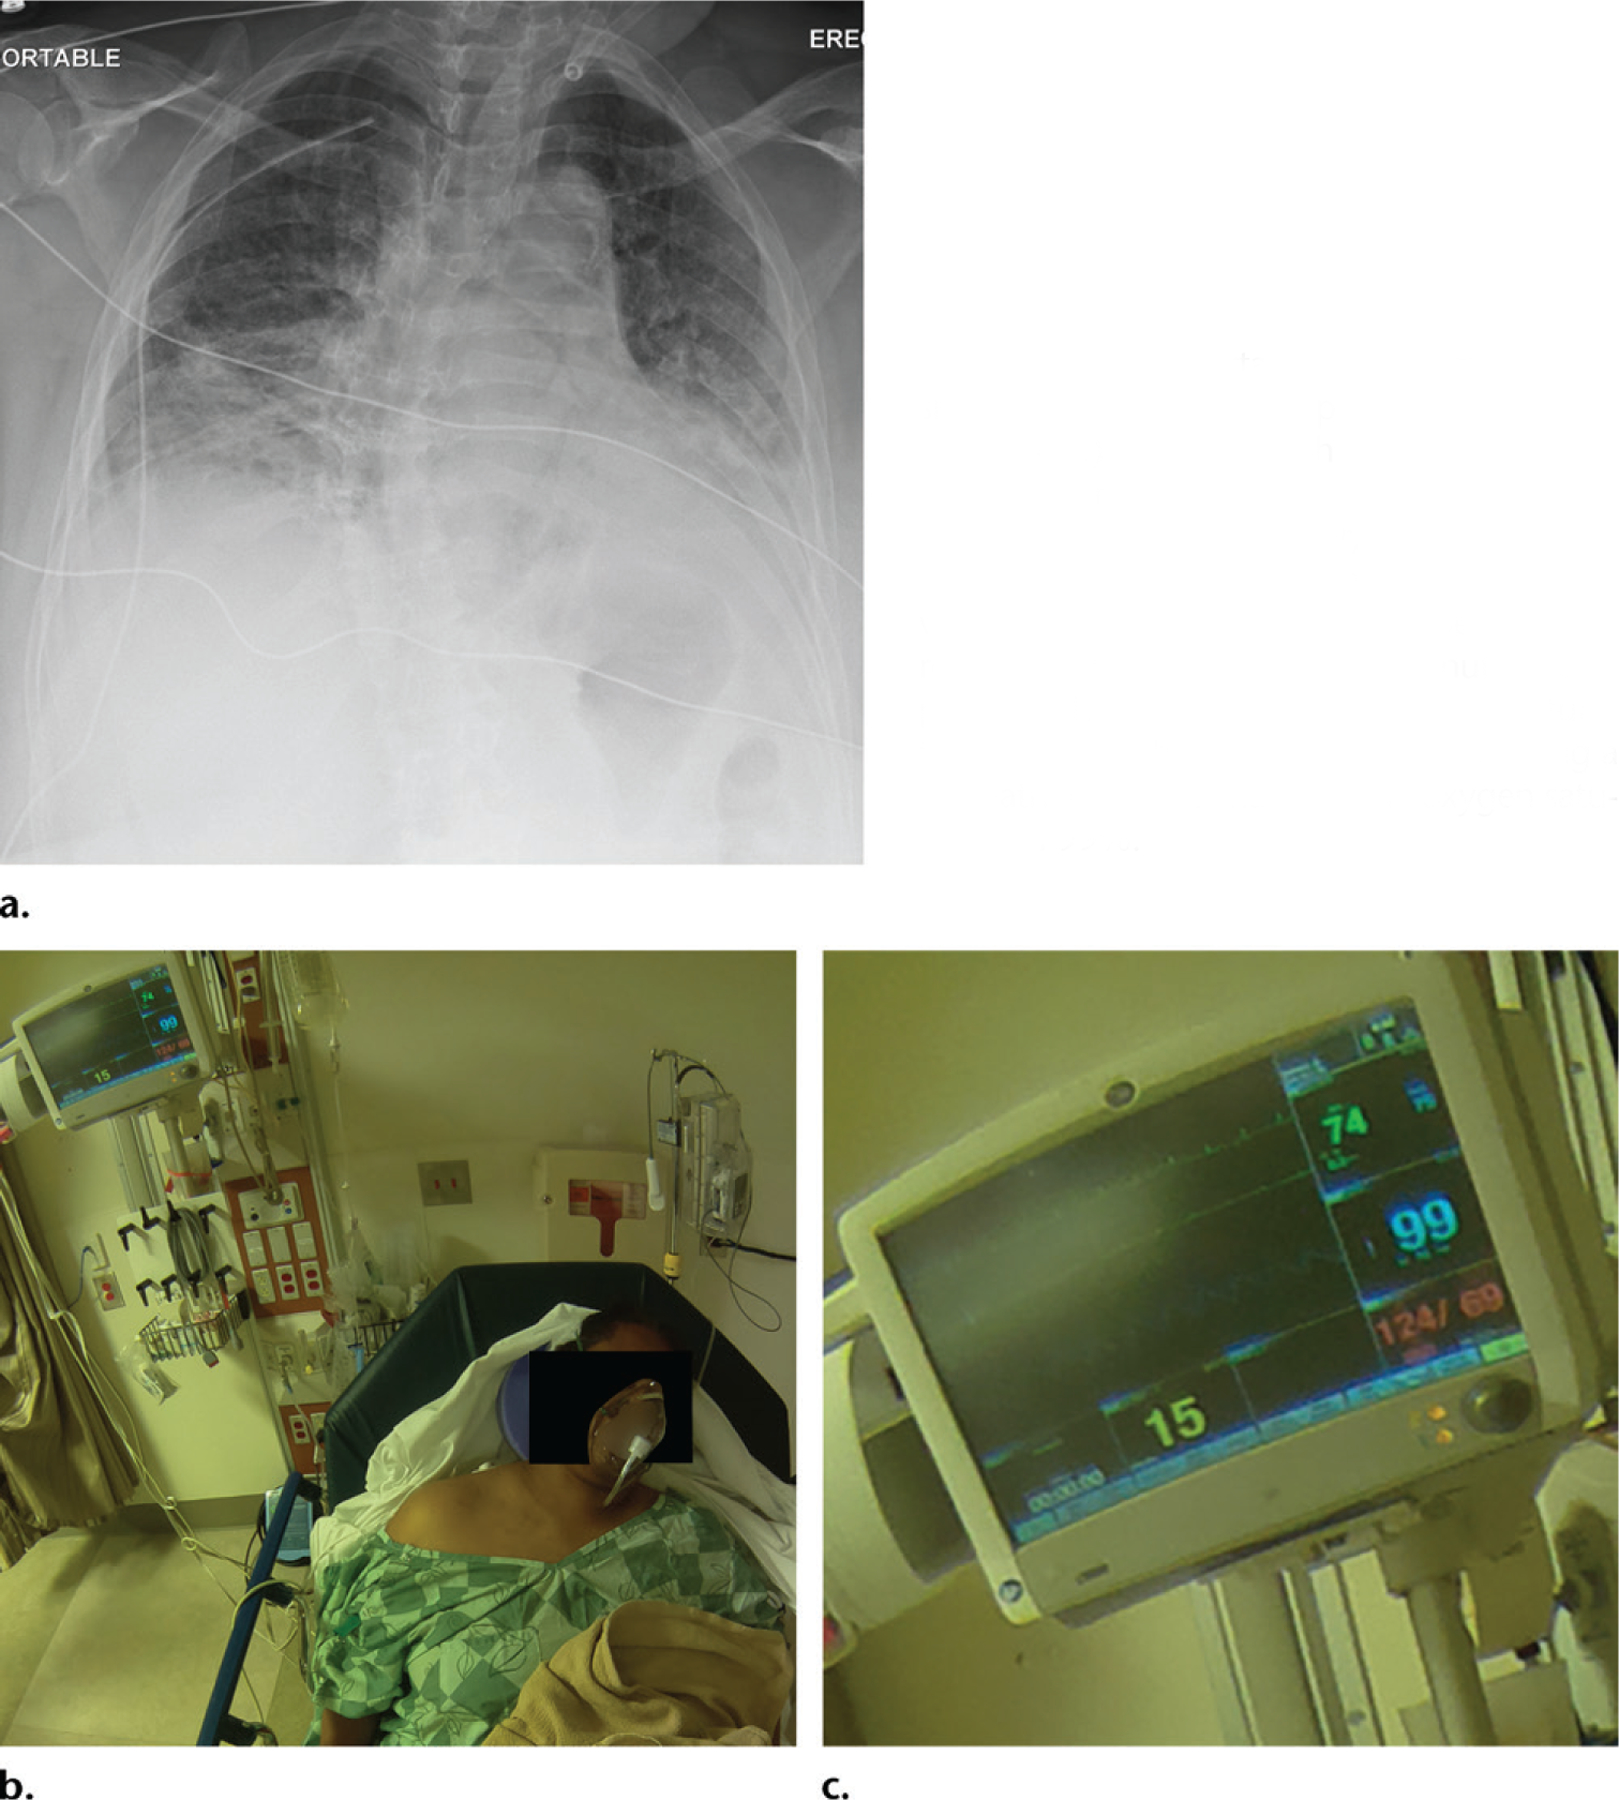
(a) Portable chest radiograph shows bibasilar airspace opacities. (b, c) Photograph (b) obtained with a wide-angle lens shows that most of the room was imaged, including the patient monitor, which has been magnified (c) to show the patient’s stable vital signs (heart rate, 74 beats per minute; respiratory rate, 15 breaths per minute; blood pressure, 124/69 mm Hg; and oxygen saturation, 99%). Note that the patient was using a rebreather mask to maintain the oxygen saturation of 99%.
